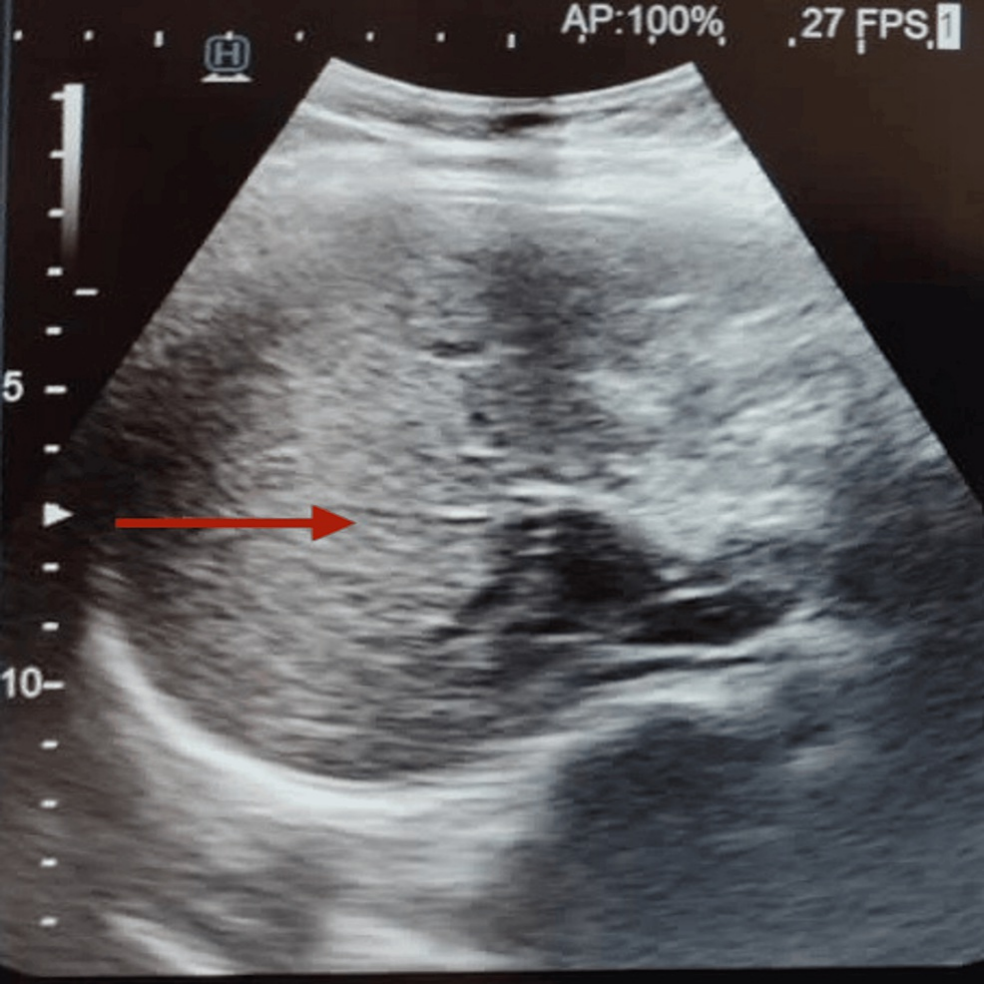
Ultrasonography of the liver with hyperechoic areas (depicted by the red arrow) suggestive of liver fibrosis.
